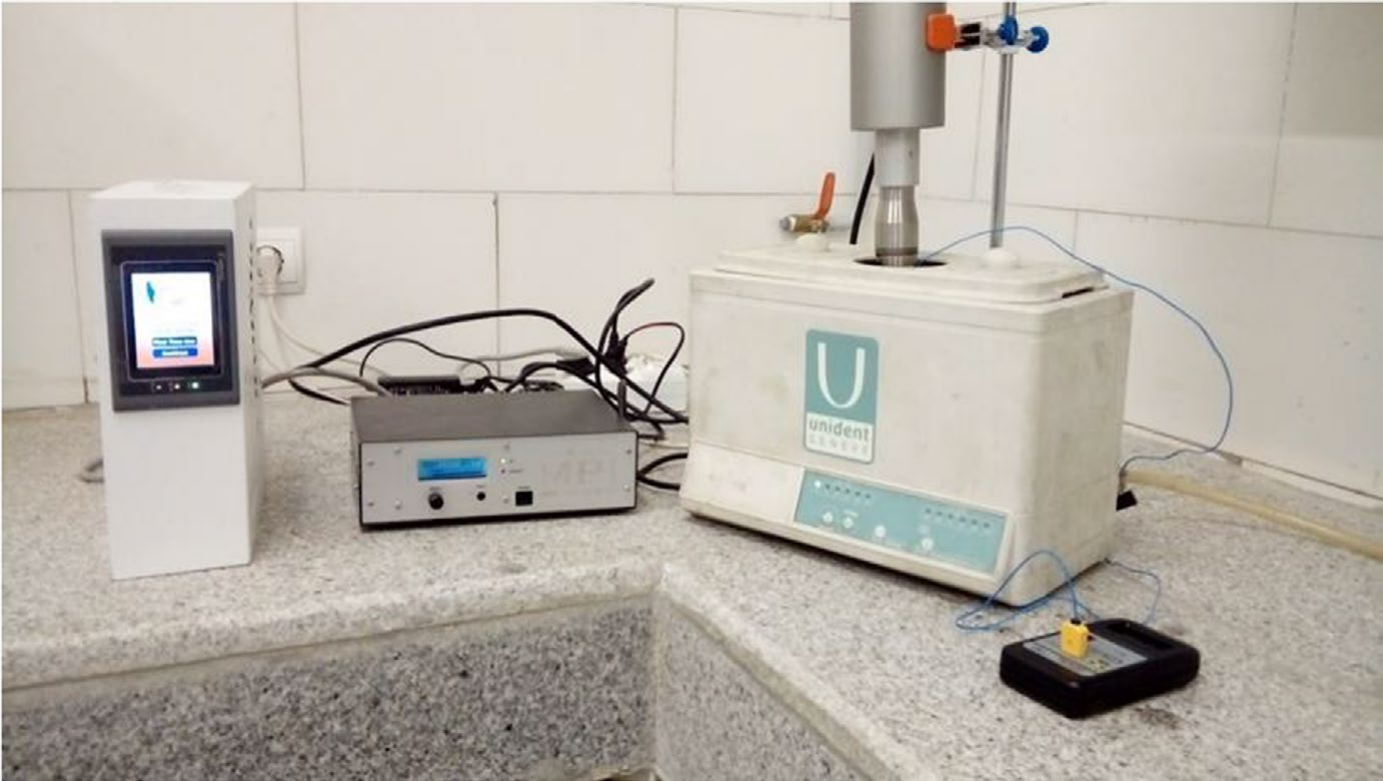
Ultrasound‐assisted extraction system
